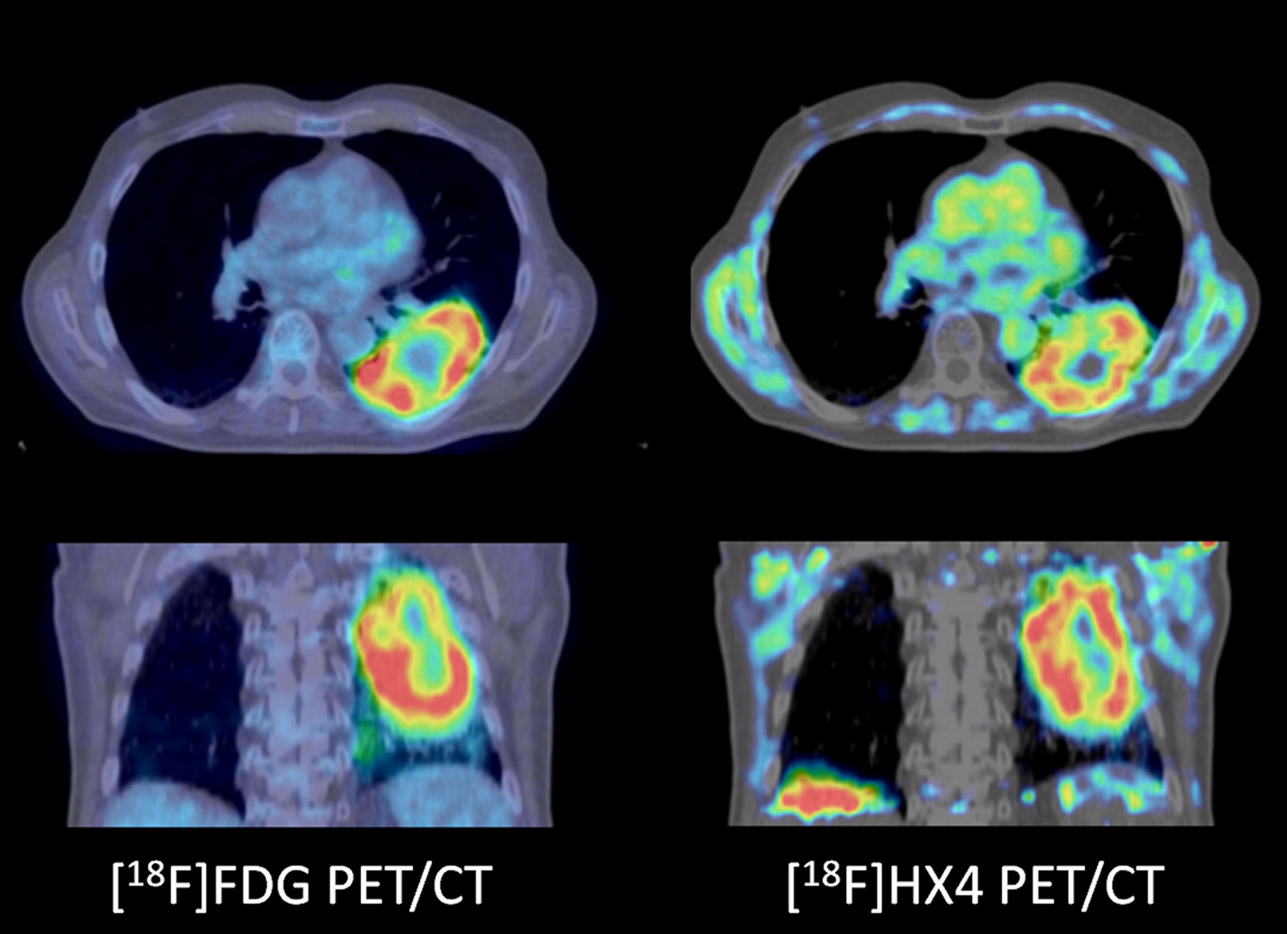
A patient with a cT3N2M0 non-small cell lung cancer not otherwise specified. PD-L1 status or molecular analyses was not performed. The tumor showed increased [^18^F]FDG uptake (left panels) as well as increased [^18^F]HX4 uptake (right panels), indicative of increased hypoxic stress. Note the regional differences of metabolic profiles in the tumor, for example the cranial part ([^18^F]HX4 more than [^18^F]FDG) versus the caudal part (both [^18^F]HX4 and [^18^F]FDG increased).
